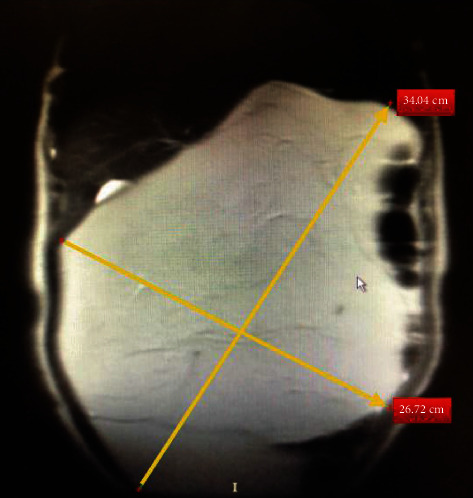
The lesion measured as approximately 34 cm × 26 cm in size on coronal view of magnetic resonance imaging.
